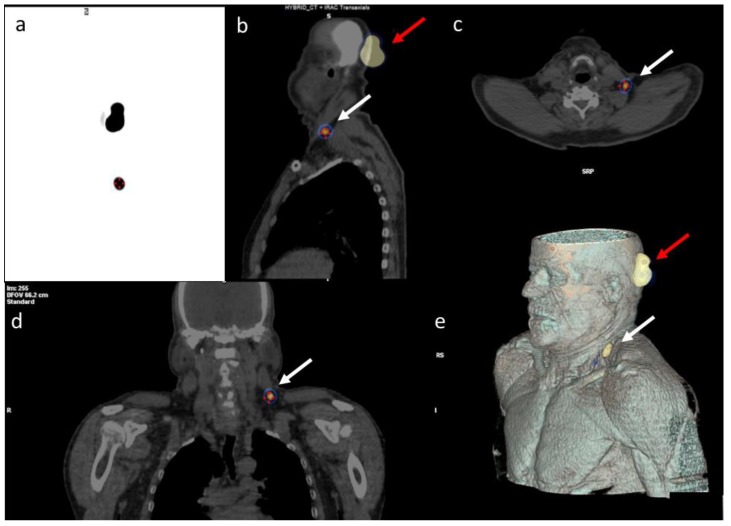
99mTc-serum albumin lymphoscintigraphy in a 64 year-old patient with a temporal region primary head and neck cutaneous melanoma. Breslow 2.5 mm. (red arrows). (a) MIP, (b) sagittal, (c) trans-axial, and (d) coronal fused images SPECT/CT. (e) A 3D reconstruction shows the sentinel lymph nodes drained to the supraclavicular region (white arrows).
